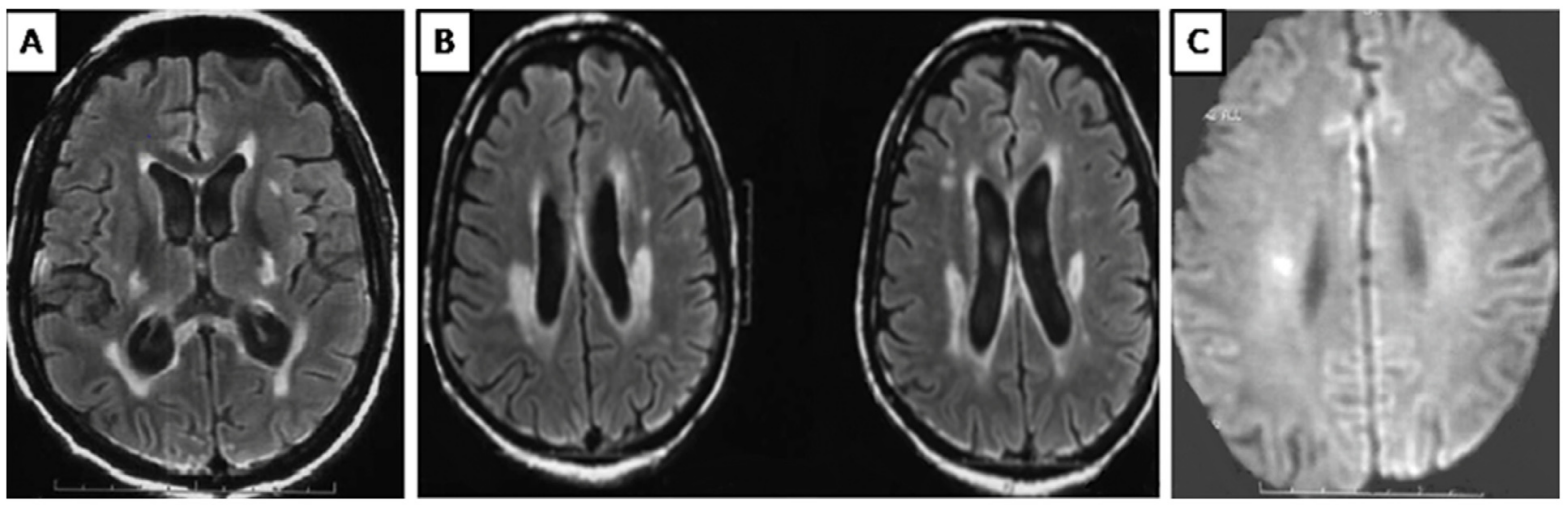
Cranial T2 FLAIR MRI axial sections in a 45- year-old male, stage III tuberculous meningitis (TBM) with type 2 diabetes mellitus and hypertension, and a 15-year-old male, stage III TBM patient. (
